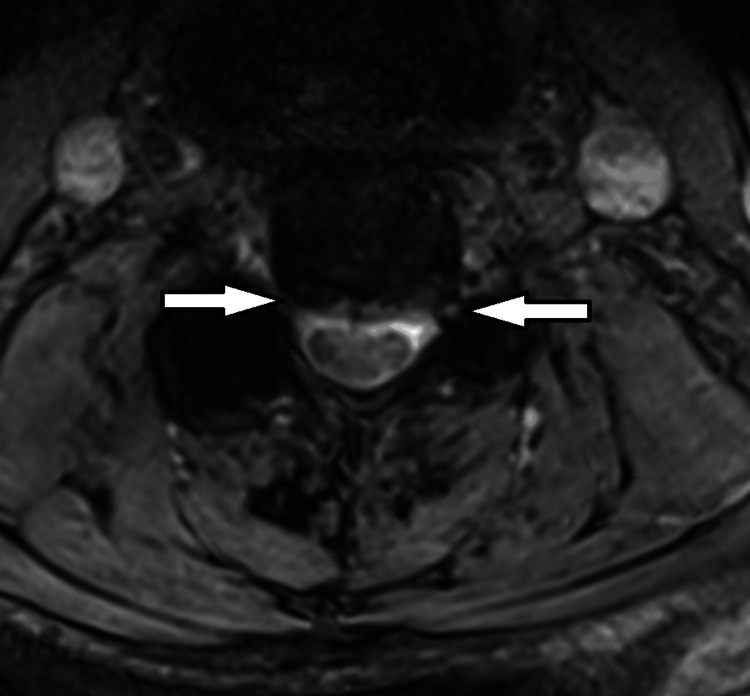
Axial T2 MRI cervical spine showing severe neural foraminal stenosis and canal narrowing at C4 (white arrows). MRI, magnetic resonance imaging.
